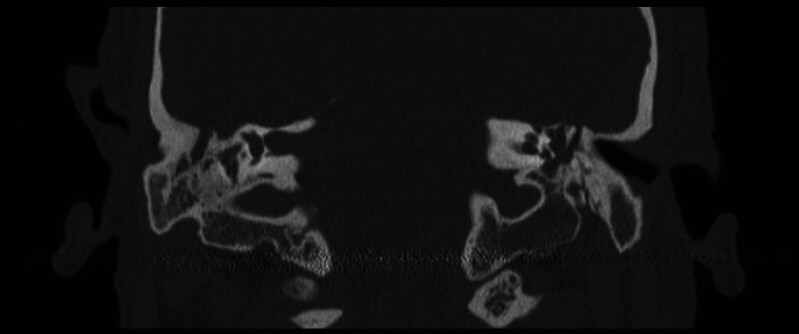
Preoperative computed tomography showing left-sided tegmen defect with the malleus at the level of the floor of the middle fossa.
